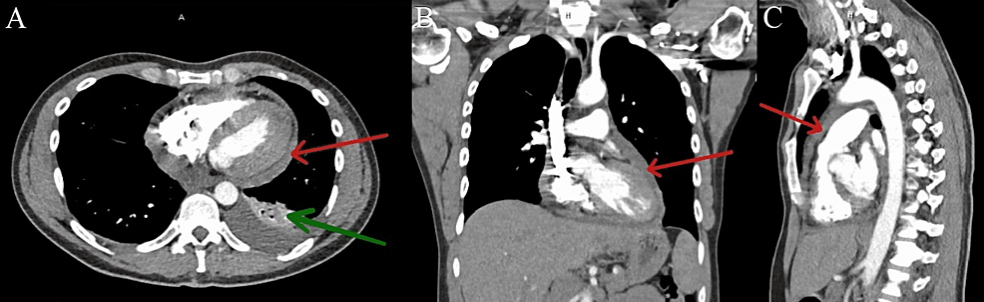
(A) CT PE protocol transverse view of thorax on January 2022. Red arrow shows small pericardial effusion and green arrow shows small left pleural effusion. (B) CT PE protocol coronal view of thorax on January 2022. Red arrow shows small pericardial effusion. (C) CT PE protocol sagittal view of thorax on January 2022. Red arrow shows small pericardial effusion.
